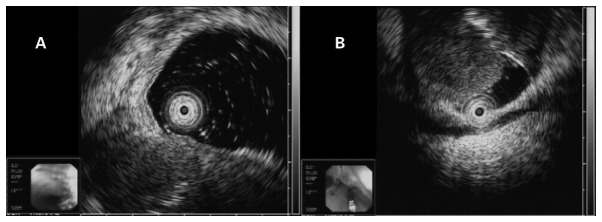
Endoscopic ultrasound. (A) In the esophagus, a hypoechoic mass with an unknown origin is revealed. (B) In the gastric corpus, a hypoechoic mass is evident.
